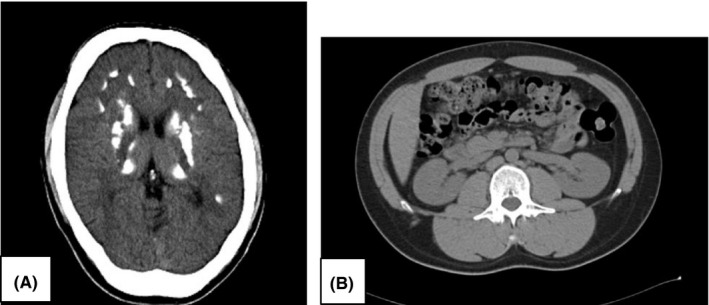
Radiographic imaging of brain, kidney. A, brain CT indicating multiple calcifications of basal nuclei. B, abdominal CT showing no obvious kidney malformation with transverse diameters of 90cm(left) and 93(right), respectively
